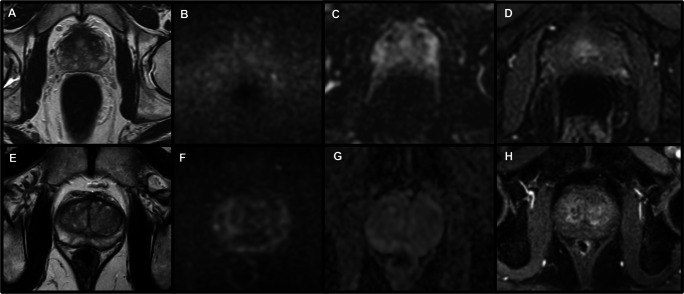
Two cases of prostate MRI from the two cohorts (pre- and post-teaching). The first case [A axial T2-weighted imaging; B diffusion-weighted imaging (high *b* value: 1,000 s/mm^2^); C apparent diffusion coefficient map; D dynamic-contrast enhanced sequences] is from the pre-teaching cohort and the reference standard was PI-QUAL 2, as only T2-WI is of sufficient diagnostic quality. Only 7/16 participants gave the correct PI-QUAL score for this scan, with 1/16 giving a PI-QUAL score of 3, 3/16 a PI-QUAL score of 4 and 5/16 a PI-QUAL score of 5. The second case [E axial T2-weighted imaging; F diffusion-weighted imaging (high *b* value: 2,000 s/mm^2^); G apparent diffusion coefficient map; H dynamic-contrast enhanced sequences] is from the post-teaching cohort and the reference standard was PI-QUAL 5. All patients scored this scan as of optimal diagnostic quality (i.e., PI-QUAL score 4 or 5; in detail: 9/16 scored PI-QUAL 4 and 7/16 scored PI-QUAL 5)
